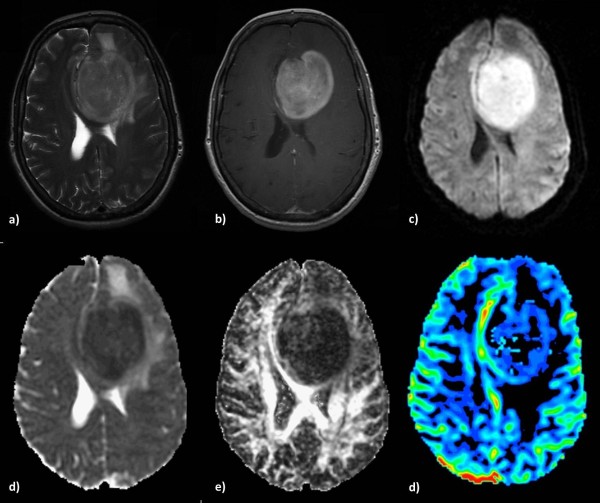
Primary Cerebral Lymphoma in a 59-year-old-woman. a) High signal intensity with peritumoral edema on a T2-weighted image, b) intense contrast-enhancement on a T1-weighted post contrast image and c) hyperintensity on a DW image. Decreased intratumoral ADC (d) and FA (e). The rCBV map shows moderate perfusion within the lesion (f).
